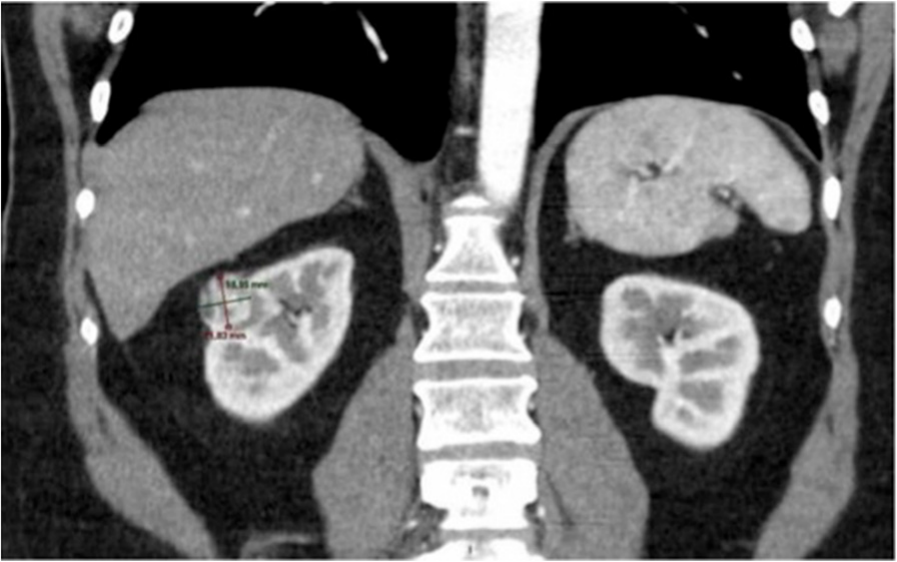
CT-scan with a 19 × 22 mm exophytic tumor of the right kidney located in the renal polar lines, 4–7 mm away from the sinus or collecting system; R.E.N.A.L.-Nephrometry Score: 7a [R(1)+E(1)+N(2)+A(a)+L(3)= 7a].
